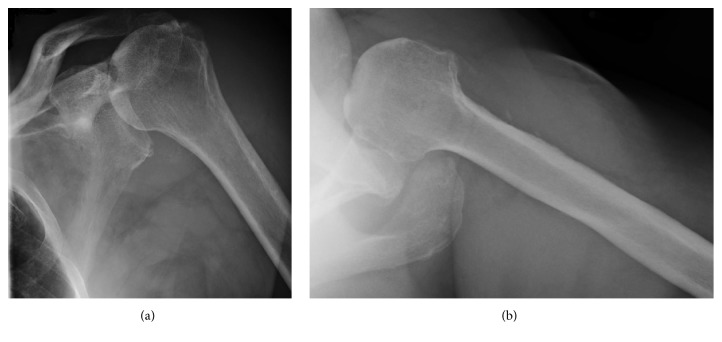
Anterior-posterior (a) and axillary lateral (b) radiographs of our patient's shoulder showing the glenohumeral dislocation.
